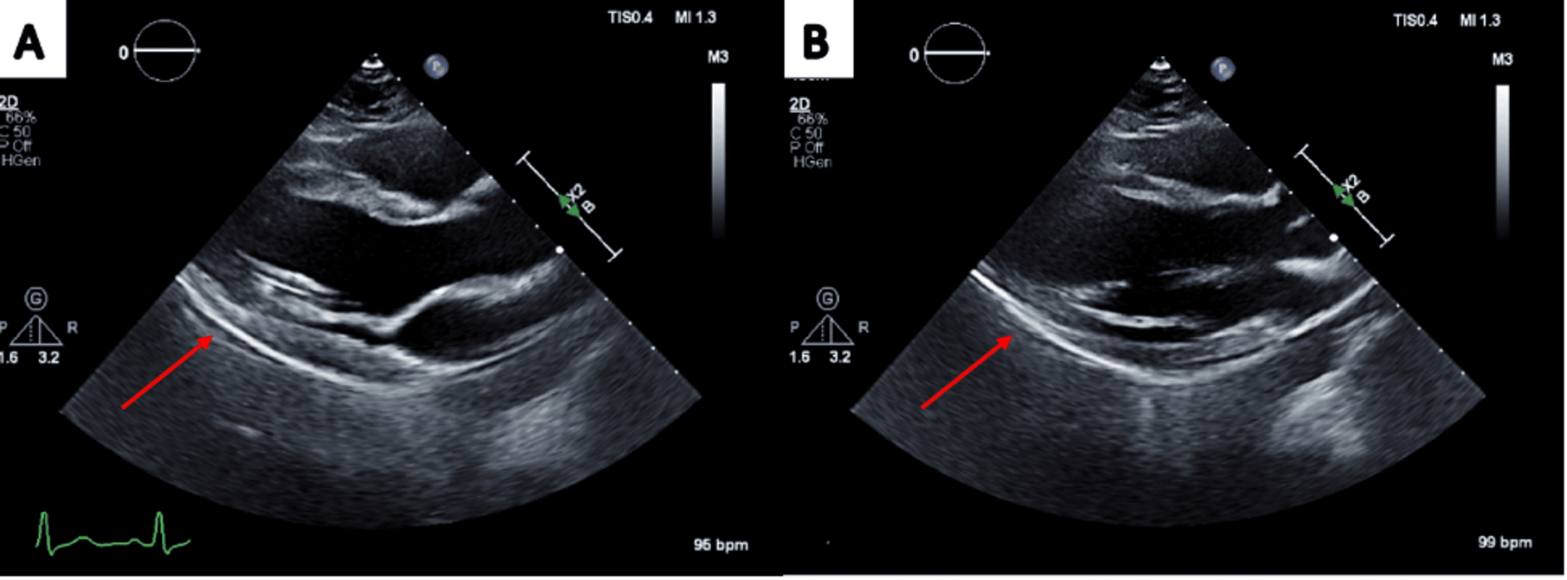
Transthoracic echocardiogram showing bright pericardium on parasternal long axis (PLAX) view (A and B) (red arrows)
